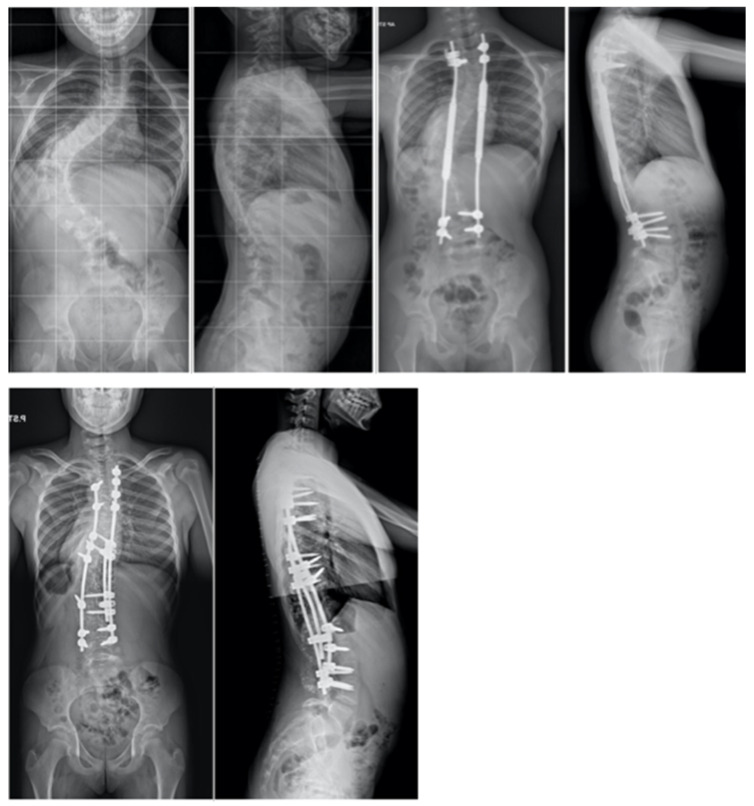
Radiographs revealed an 11-year-old female with severe early onset idiopathic scoliosis and asymptomatic spondylolisthesis. At the age of four, she underwent a four-level anterior release using a mini-open anterior approach, followed by the placement of magnetically controlled growing rods (MCGRs) through a less-invasive technique and subsequent periodic ambulatory lengthening. When she turned 11, she underwent a procedure involving conversion to posterior spinal fusion, replacement of MCGRs, and multi-level posterior release with Ponte osteotomy.
